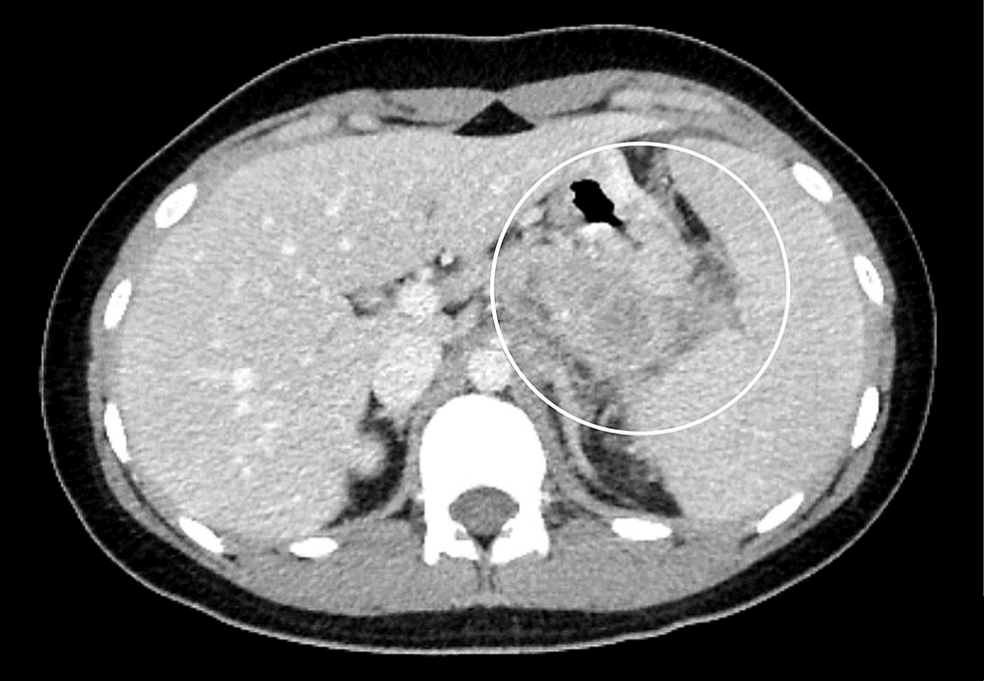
Abdominal CT revealed pancreatic and splenic arteriovenous infiltration (circle).
